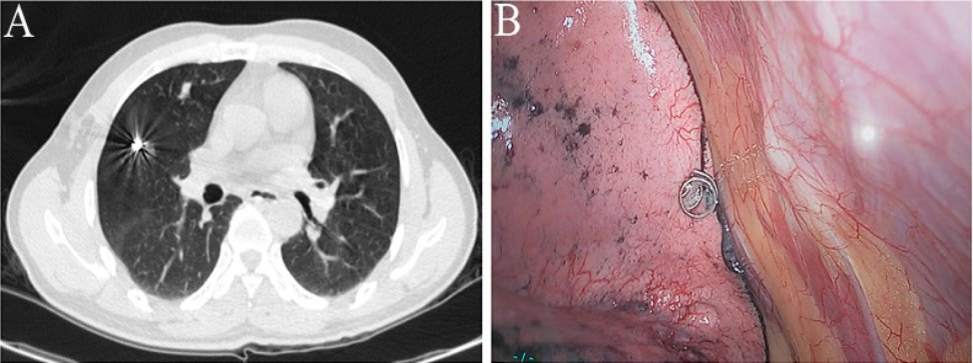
Images of lung CT scan and surgical field for coil localisation. (A) A GGN in the right middle lobe was localised with the coil. (B) The coil for lesion localisation was found in the upper lobe
